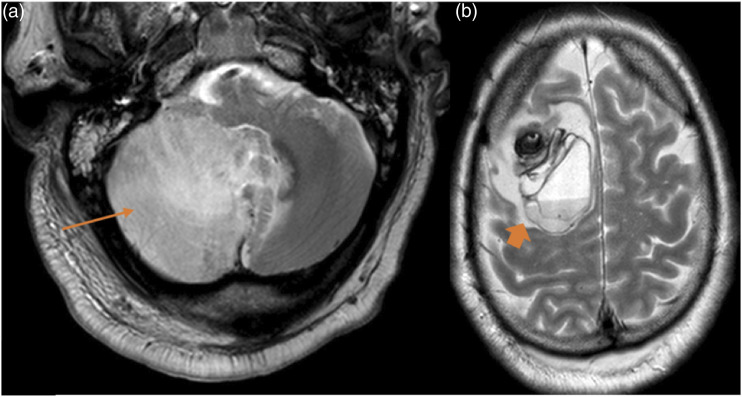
Ischaemic infarct and intraparenchymal haemorrhage. A 53-year-old male patient with usual interstitial pneumonia underwent double-LT. Five days after the transplantation, he presented a decreased level of consciousness. Figures: axial T2-weighted sequences (a and b). Right cerebellum infarction (thin arrow) with IV ventricle compression. Right frontal intraparenchymal haemorrhage (thick arrow) near to the derivation valve-shunt.
